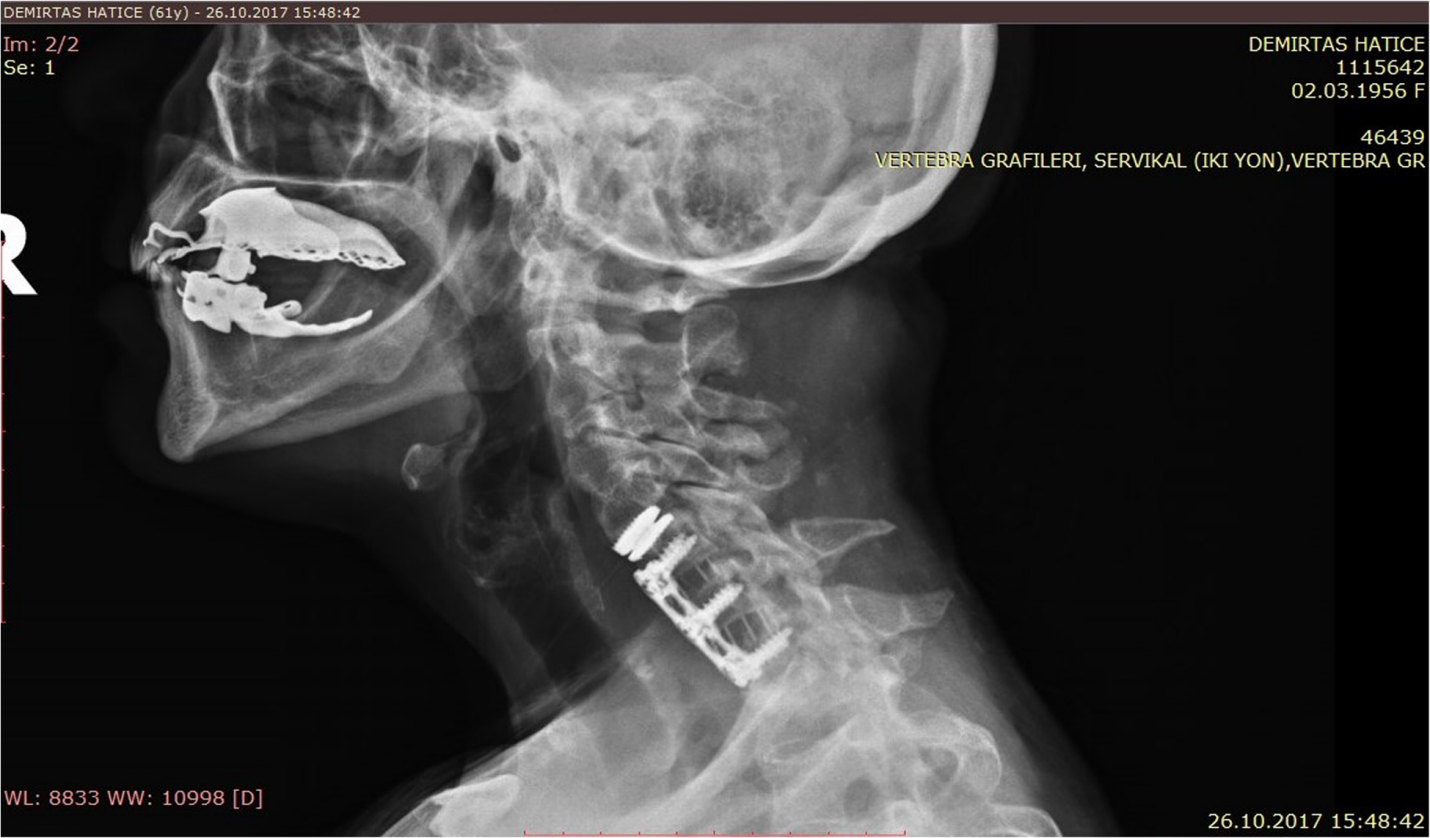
Plain radiograph demonstrating hybrid construction with disc prosthesis for C4–C5 and anterior fusion for C5–C6, C6–C7
